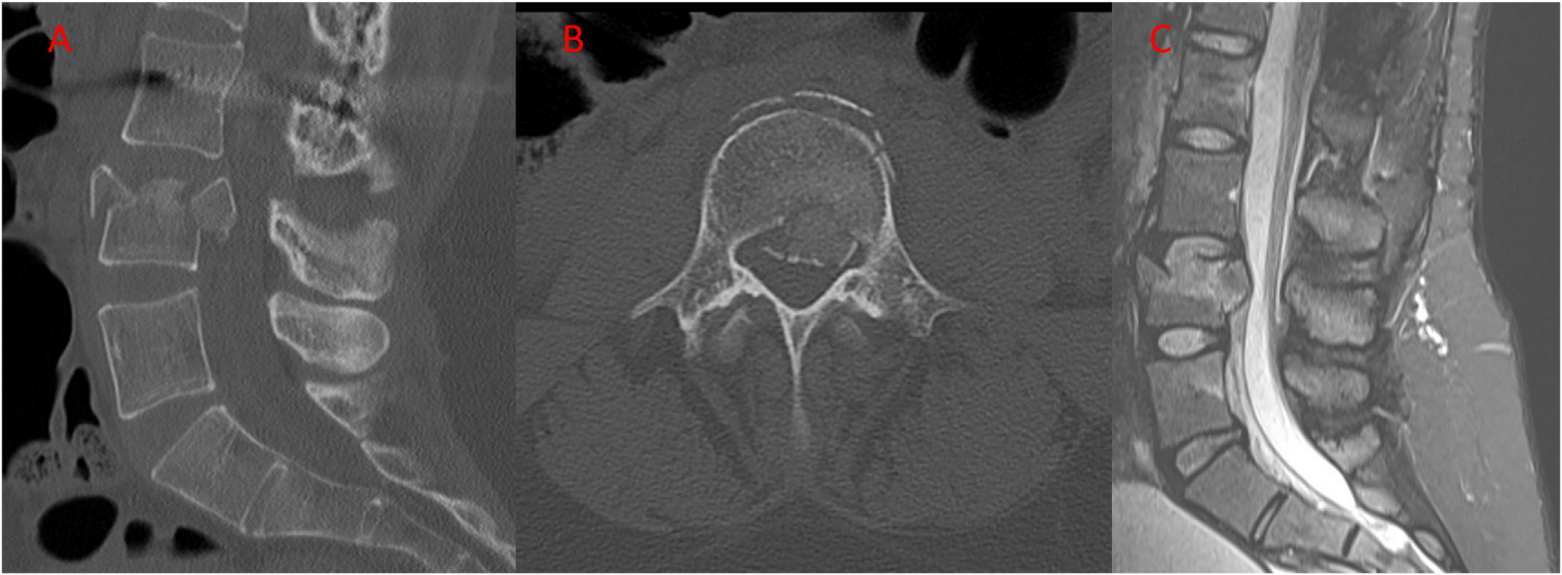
Sagittal (A) and axial (B) CT scan images showing an L4 burst fracture with central narrowing demonstrated by the sagittal MRI image (C).
